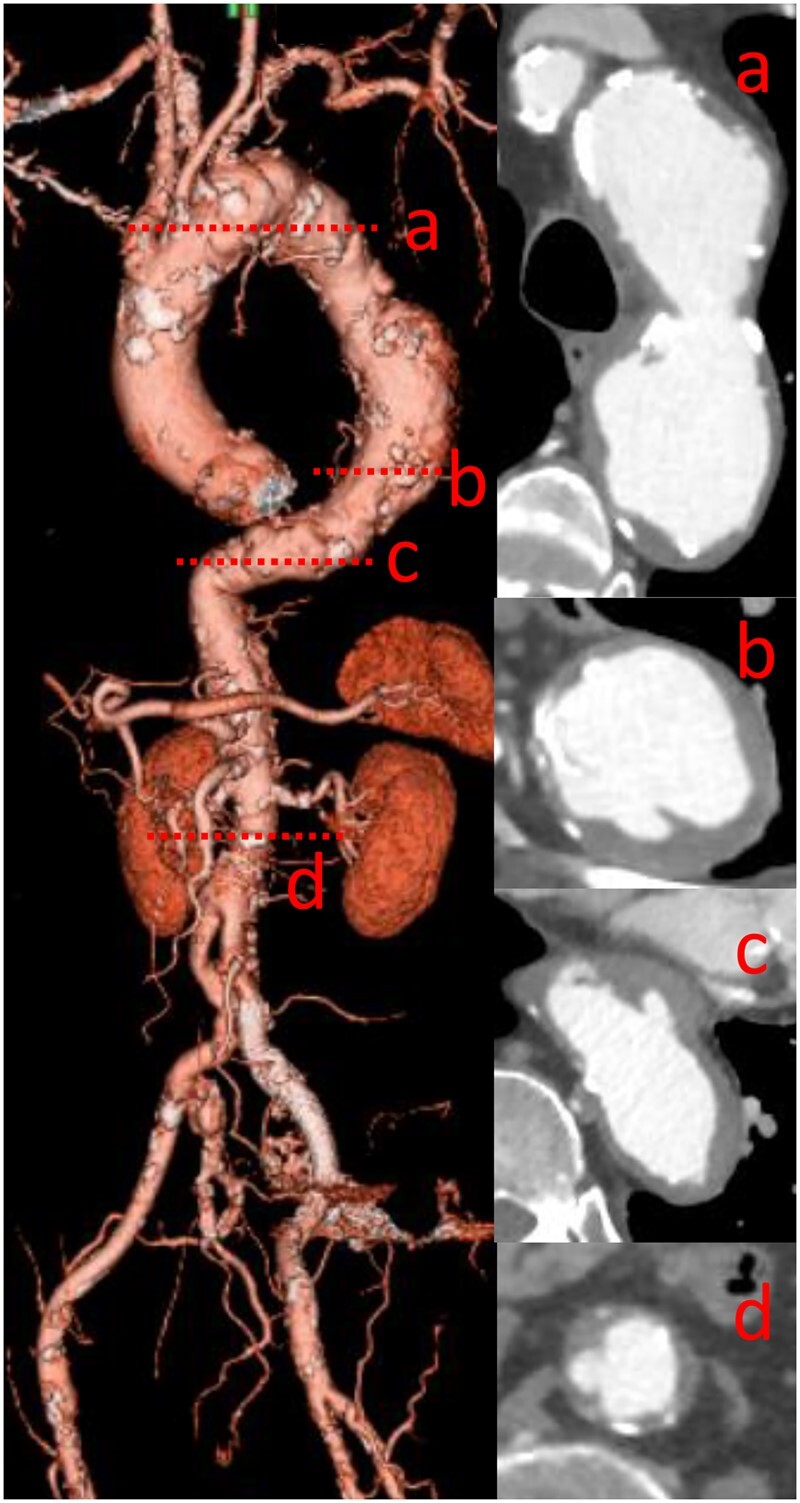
Shaggy aorta. The aortic images on computed tomography angiography of the patients with the typical case of shaggy aorta is showing; the red dotted lines from (a) to (d) indicate the location of each cross-section.
